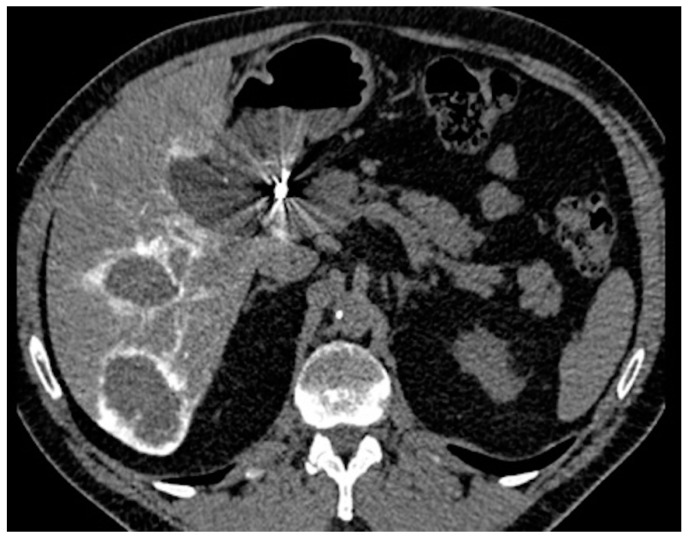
CT scan with contrast injected through the arterial port catheter.
